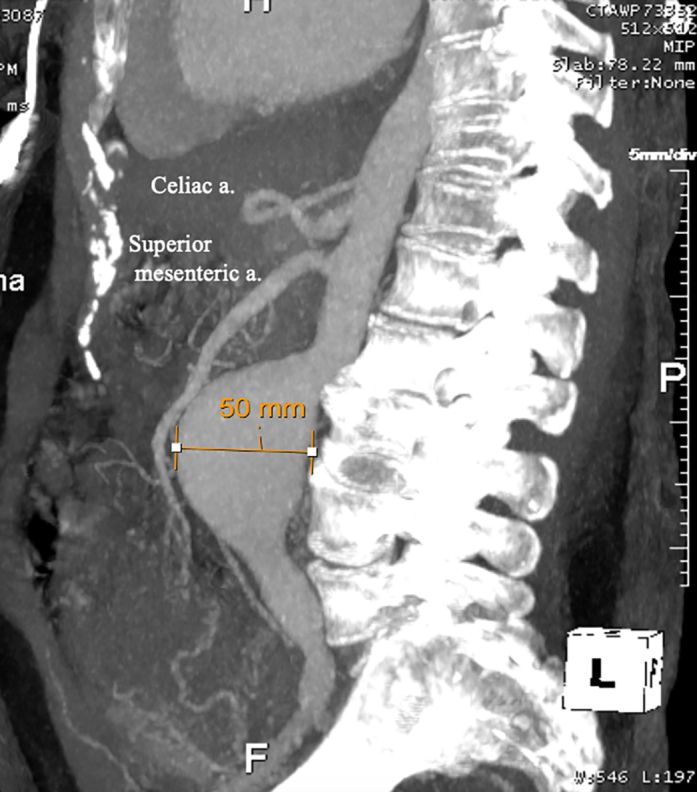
CTA on Postpartum Day 2 Demonstrating Rapid Growth of the Infrarenal Aorta to 5.0 cm CTA = computed tomography angiography.
